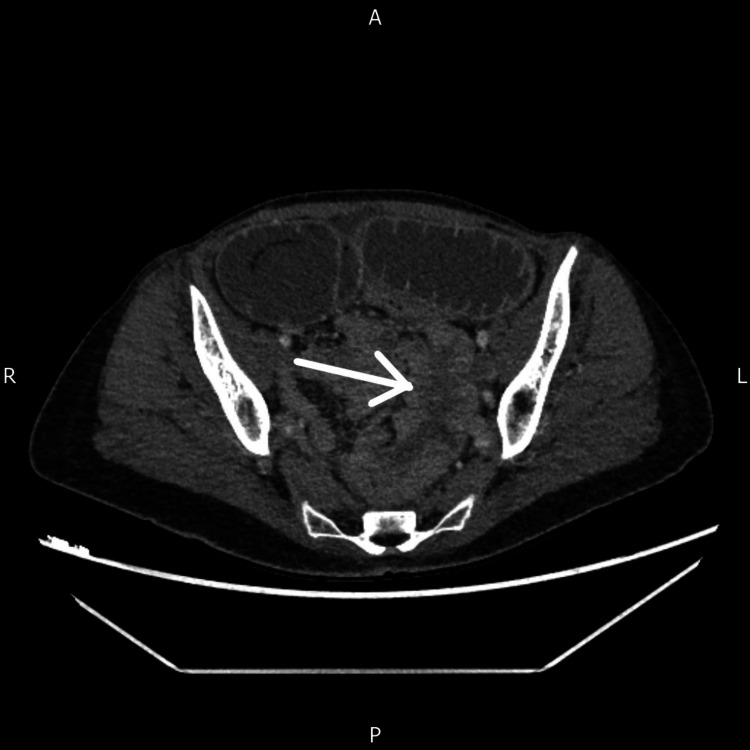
CT scan of the abdomen and pelvis showing a small bowel intussusception as observed in the axial view CT: computed tomography
